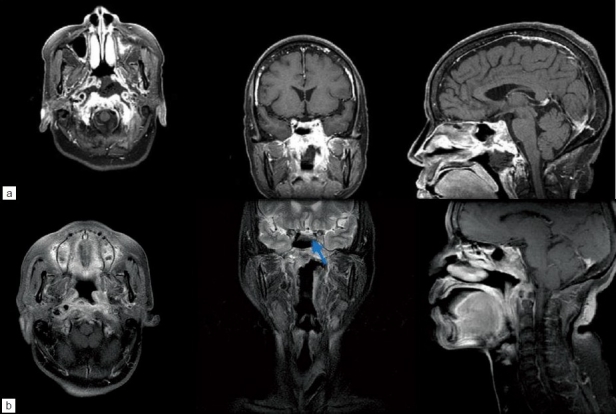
(a) MRI scan of sella. Preoperative axial, coronal and sagittal images after intravenous administration of gadolinium diethylenetriamine penta-acetic acid are shown. Thickening of the nasopharyngeal wall with irregular contrast enhancement is seen. Ill-defined enhancement over the skull base including the clivus, bilateral petrosal apex and condylar process of the occipital bone is also seen. Prominent enhancement over the left cavernous sinus and left parasellar region can also been seen. (b) Two months postoperative axial, coronal, and sagittal postgadolinium images show improvement. Residual left sixth nerve palsy was presented after the surgery, while other cranial neurolopathies improved. There were some residual abscesses in the left cavernous sinus and parasellar region (arrow)
